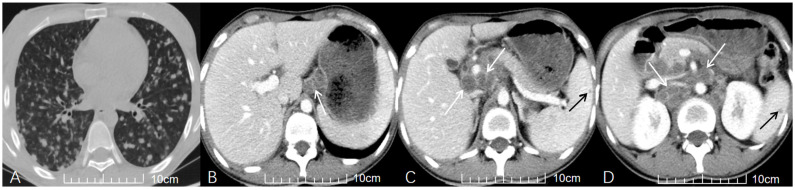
A young HIV-positive patient with recurrent fever for one month. (A) Axial non-contrast chest CT reveals subacute hematogenous disseminated PTB, manifesting as diffuse nodules (2–5 mm) in both lungs. (B–D) Axial contrast-enhanced abdominal CT (venous phase) demonstrates multiple enlarged lymph nodes in the hepatoduodenal ligament, portacaval space, and upper para-aortic region, exhibiting peripheral rim enhancement and fusion (white arrows in (B), (C), (D), respectively). Concurrent splenic TB is indicated by the presence of low-attenuation nodules (black arrows in (C,D)).
